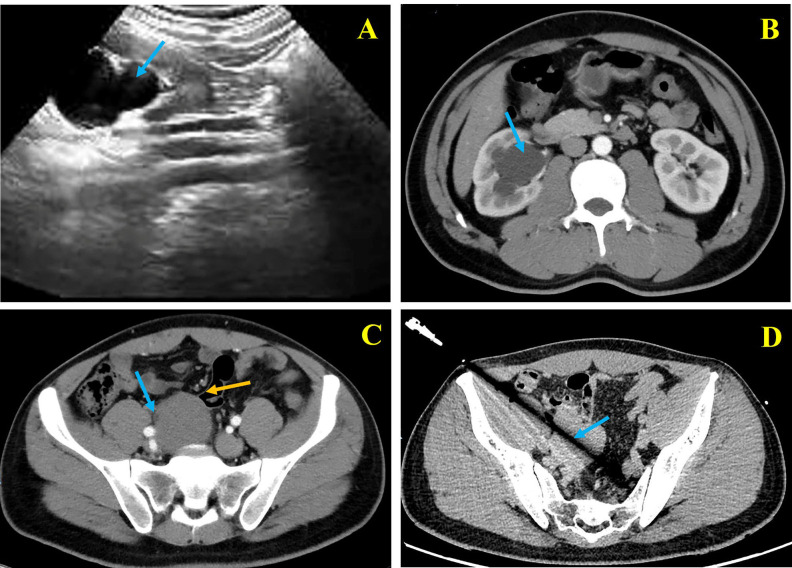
Preoperative imaging of the patient. (A) (ultrasound) and (B) (CT) showing right hydronephrosis (blue arrow). (C) Contrast-enhanced CT demonstrating right pelvic mass encasing distal ureter (blue arrow) with indistinct margins with adjacent small bowel (yellow arrow). (D) CT-guided core biopsy in tumor (blue arrow).
